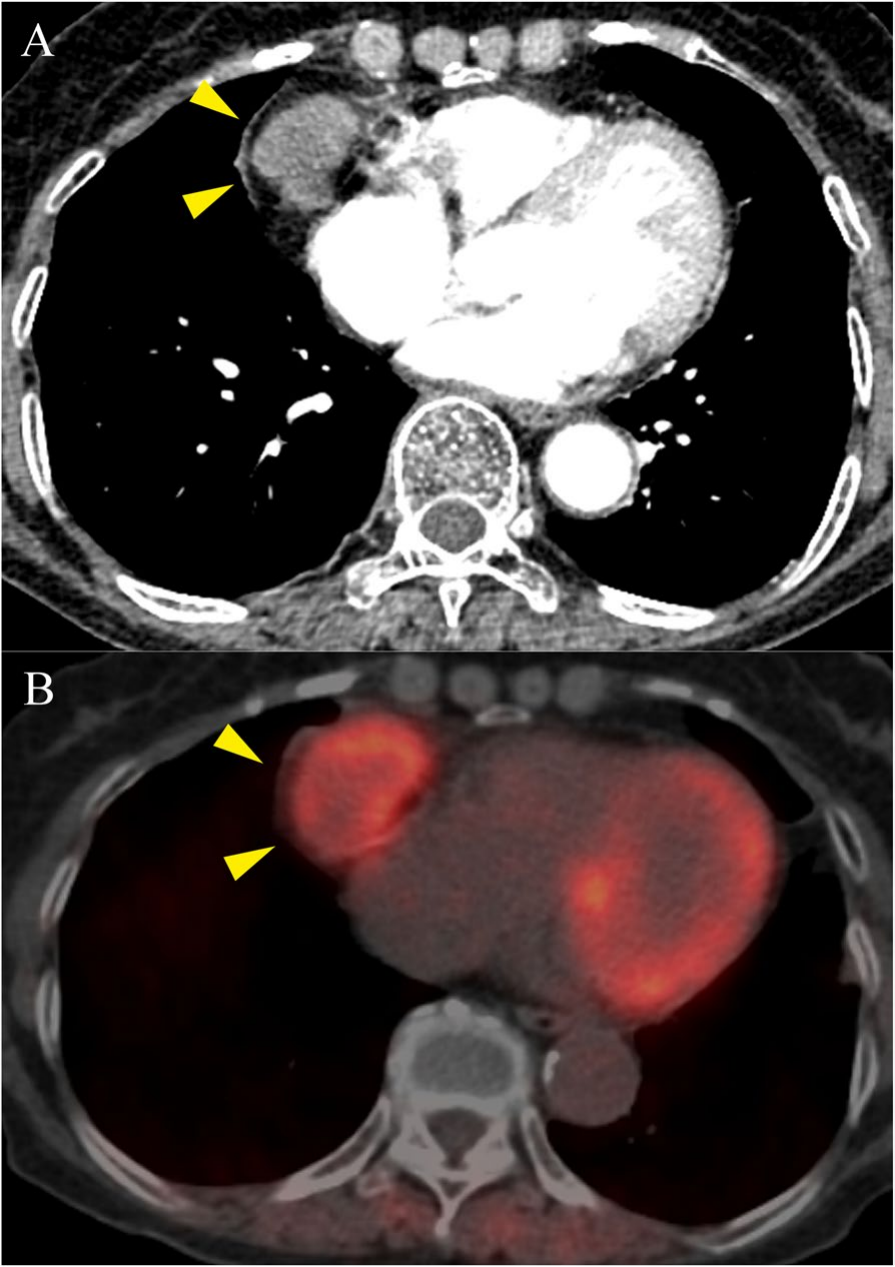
A Chest contrast-enhanced computed tomography image showing an enhanced heterogeneous mass, sized 4.5 cm, in the mediastinum. B FDG positron emission tomography revealed abnormal FDG uptake (SUVmax = 6.31) in the mass. Yellow triangles in A and B indicate the tumor
